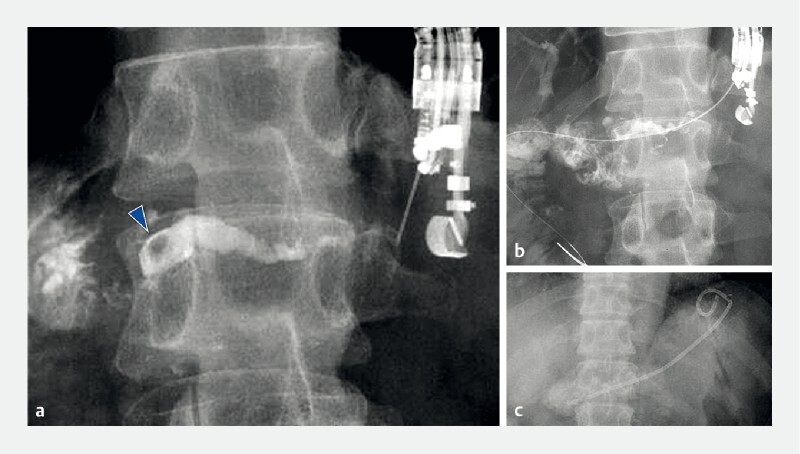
Previous endoscopic ultrasound-guided pancreatic duct drainage.
